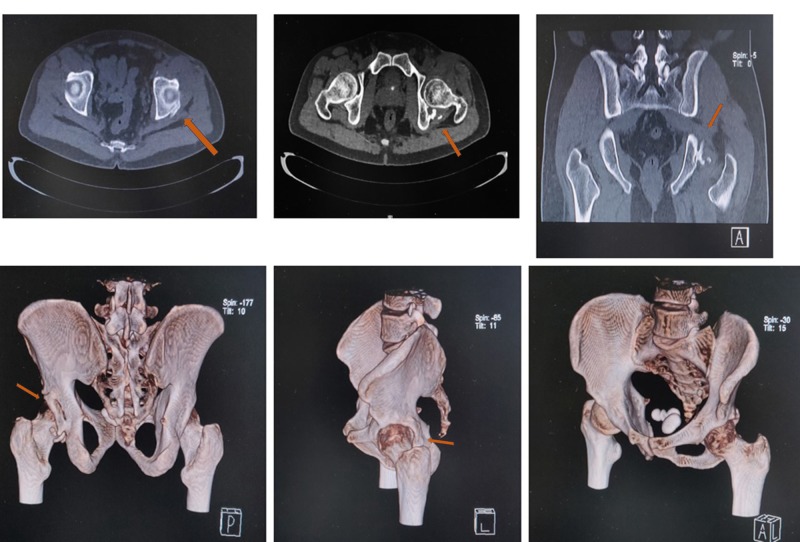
CT imaging at one year Coronal, axial, and three-dimensional (3D) section of the computed tomography (CT) scan at one year, showing the complete bony union of the anterior and posterior walls of the acetabulum
